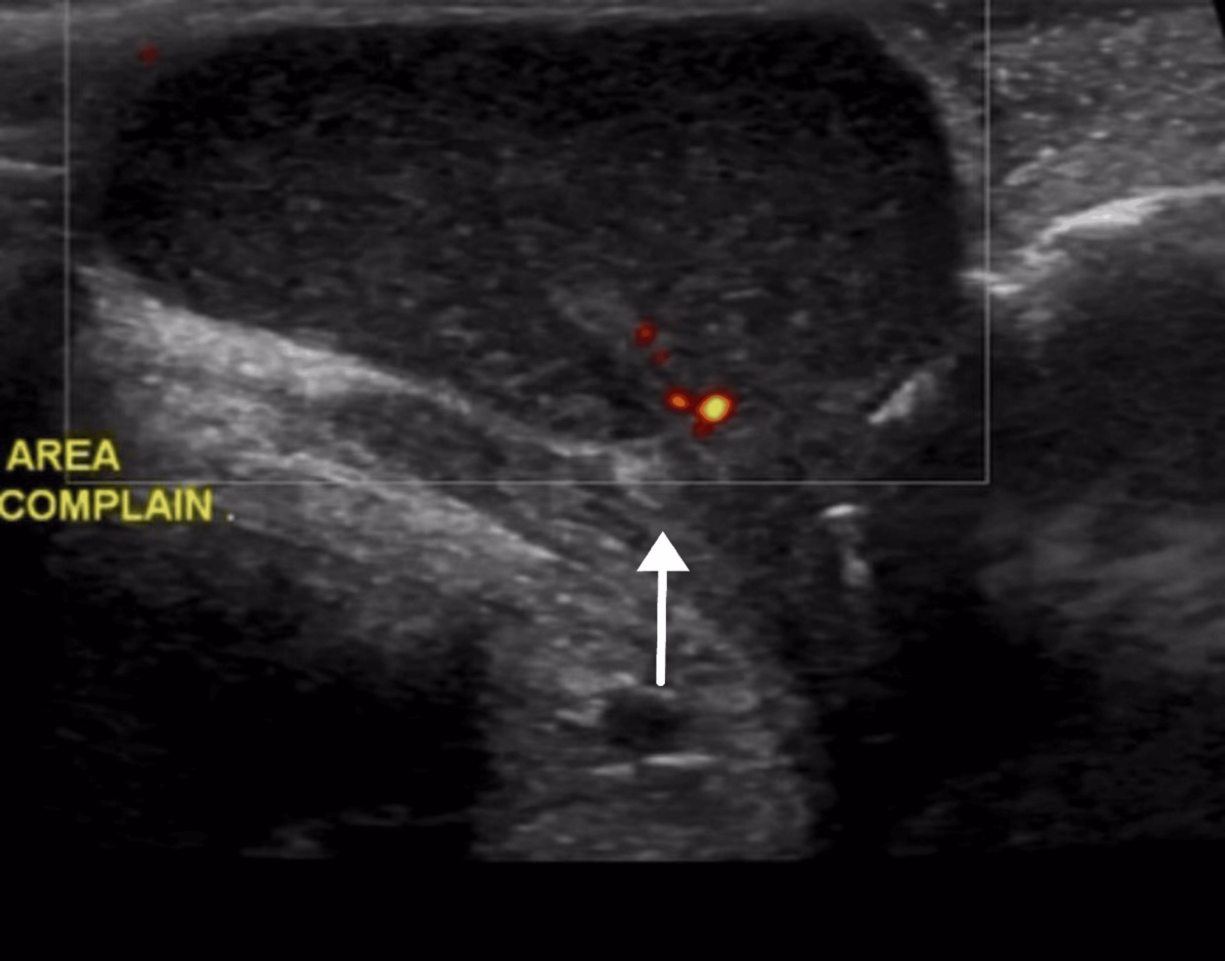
Color Doppler shows no Doppler signal.
